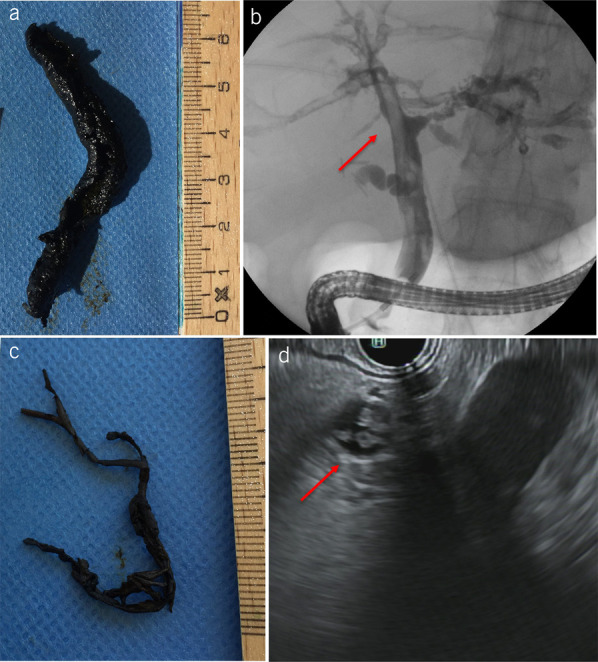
Biliary casts I. (a and c) Extracted casts of different shapes. (b) endoscopic retrograde cholangiopancreatography shows biliary casts as ribbon-like intraductal filling defects (arrow) (d) Radial endoscopic ultrasound (EUS) of the common bile duct shows biliary casts as hyperechogenic material (arrow). (c has been adapted from Leonhardt et al. doi: 10.1007/s00134-023-07257-8.)
